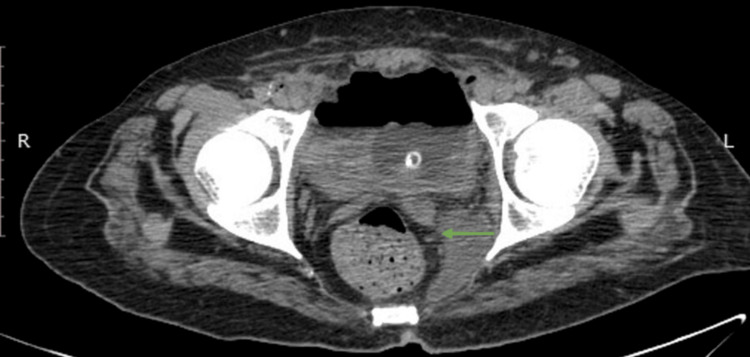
Non-contrast CT KUB showing multiple intramural gas foci within the bladder wall (arrow), with focal anterior bladder wall dehiscence and surrounding perivesical fat stranding. CT KUB: Computed tomography of the kidney, ureter, and bladder.
